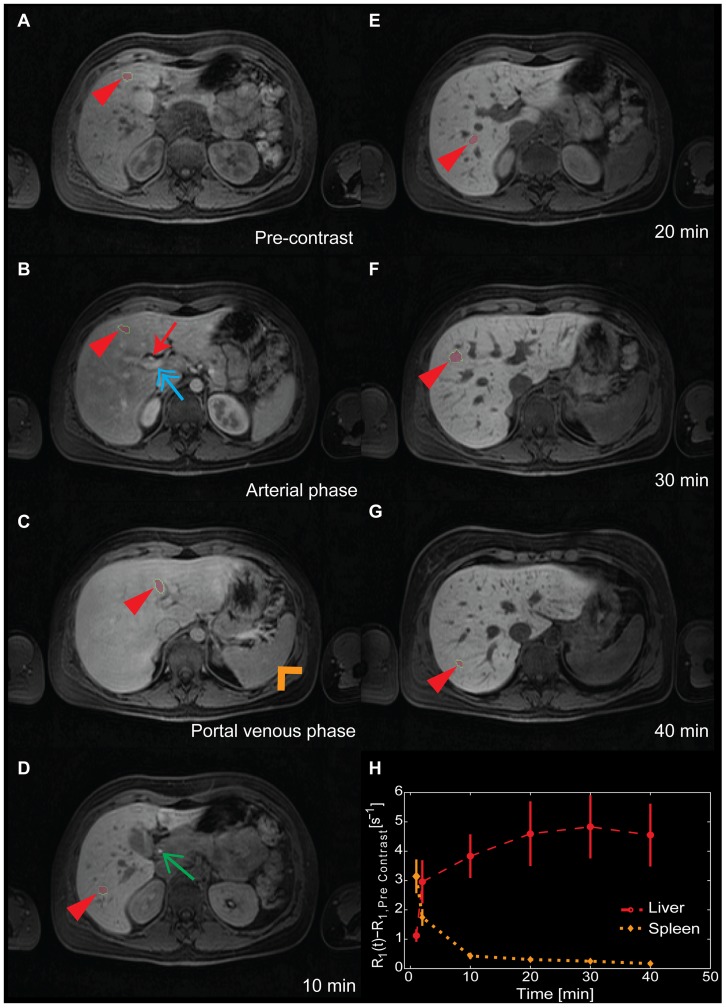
Estimation data. Example of the spatially highly resolved DCE-MRI that was used as estimation data, showing the pre-contrast acquisition (panel A), followed by the post-contrast acquisitions (panels B–G) with a distinct accumulation of the contrast agent in the liver. The dark areas within the liver in the late phases (panels D–G) are mainly blood vessels with drastically lower concentration of contrast agent compared to the accumulated contrast agent in the hepatocytes. This difference can be appreciated quantitatively in panel H, since the increase in signal intensity in the spleen is only due to the CA residing within the blood plasma. The location of the seven different regions of interest placed in the liver are shown throughout the time series (panels A–G, one in each panel), indicated by the red arrow heads. The hepatic artery, with a high initial concentration of contrast, can be seen in panel B (red arrow) as well as the portal vein (blue double arrow). In panel C the spleen (orange open arrow head) is almost isointense with the liver. The efflux of CA in the common bile duct can be seen in panel D (green arrow). Finally, the quantified mean relative change in relaxivity in the ROIs placed in the liver and spleen throughout the examination is shown in panel H, with the vertical bars corresponding to the standard error of the mean.
